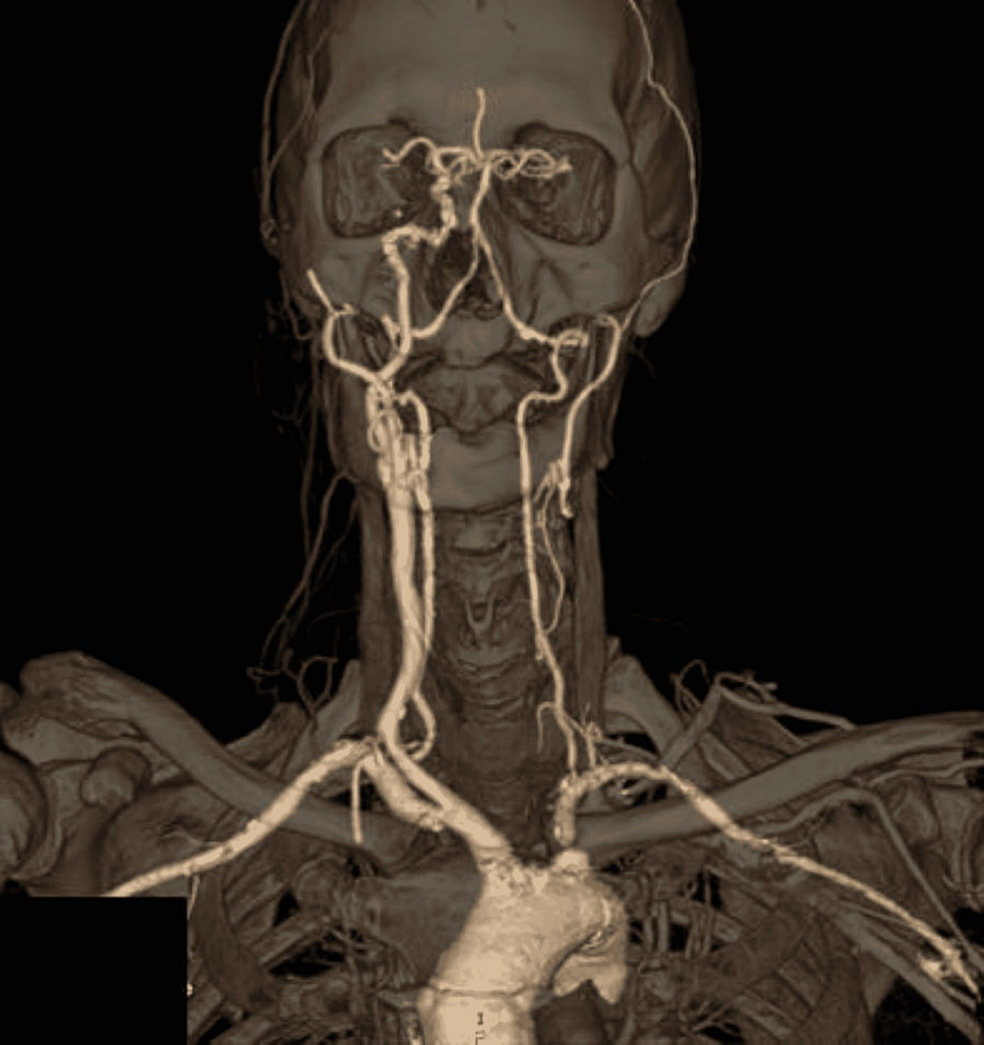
3D head and neck CTA reconstruction (anterior view).
